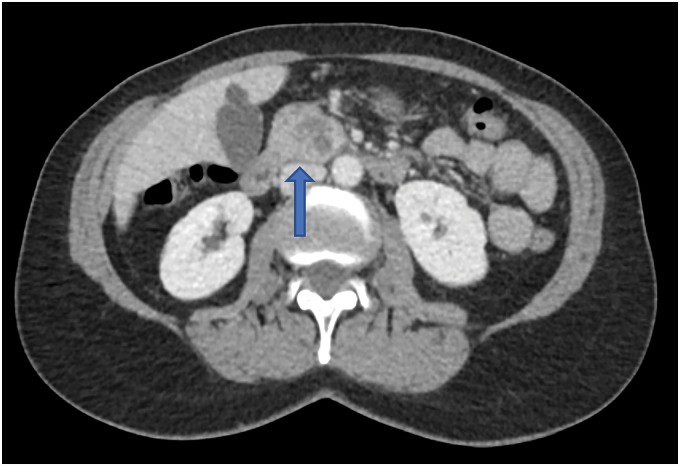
Axial CT scan of the abdomen in case 1 with intravenous contrast revealing a complex 2.3 cm pancreatic head lesion (arrow). CT, computed tomography.
